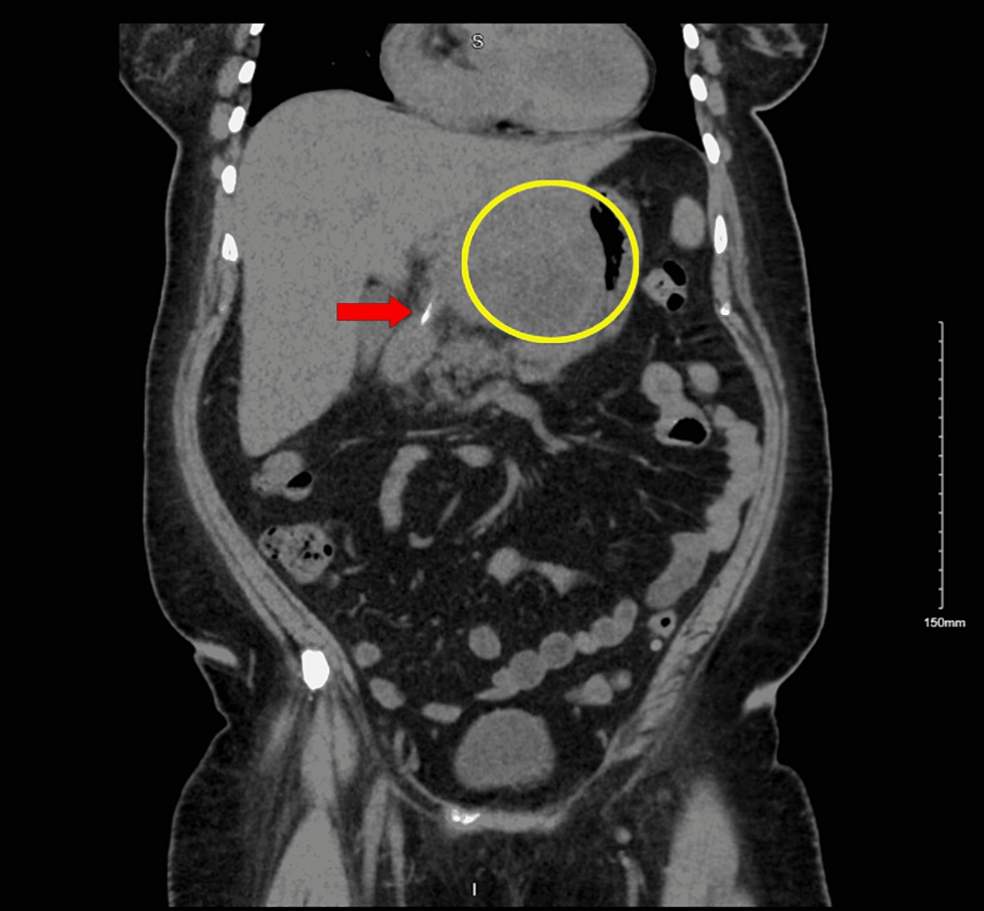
Initial CT of the abdomen and pelvis with contrast, coronal view Impressions: 1) There is an 8 x 8 x 10 cm irregular enhancing hypodense lesion occupying a large portion of the left hepatic lobe. Multiple gas pockets are also present. This finding is concerning for intrahepatic abscesses. 2) 3-mm linear hyperdensity in the region of the duodenal bulb/pylorus that appears to extend through the superior wall into the left hepatic lobe. This may be an ingested foreign body with perforation, causing the intrahepatic abscess. 3) Layering debris in the gallbladder suggestive of stones/sludge CT: computed tomography
